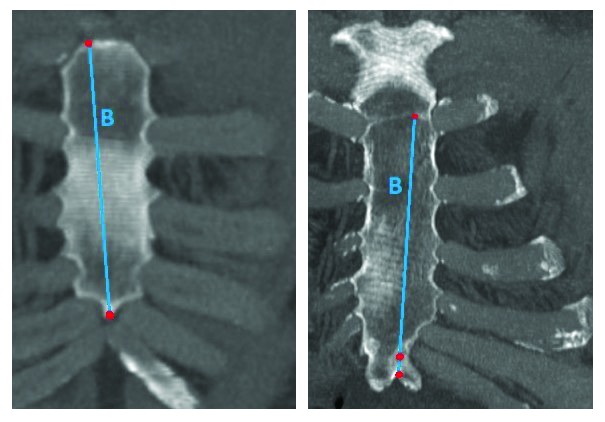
The multi-slice computed tomography (MSCT) images of two sternal bodies showing exemplary situations for landmark recognition. MSCT scan on the left allowed easy identification of landmarks for sternal body length (B), while the right one has fewer clear contours.
